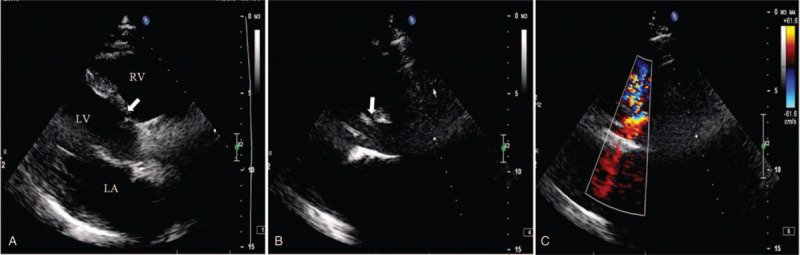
Two-dimensional Trans-thoracic parasternal long-axis view showing the ventricular septal defect (VSD) shunt from left to right. (LA = left atrium; LV = left ventricle; RA = right atrium; RV = right ventricle).
